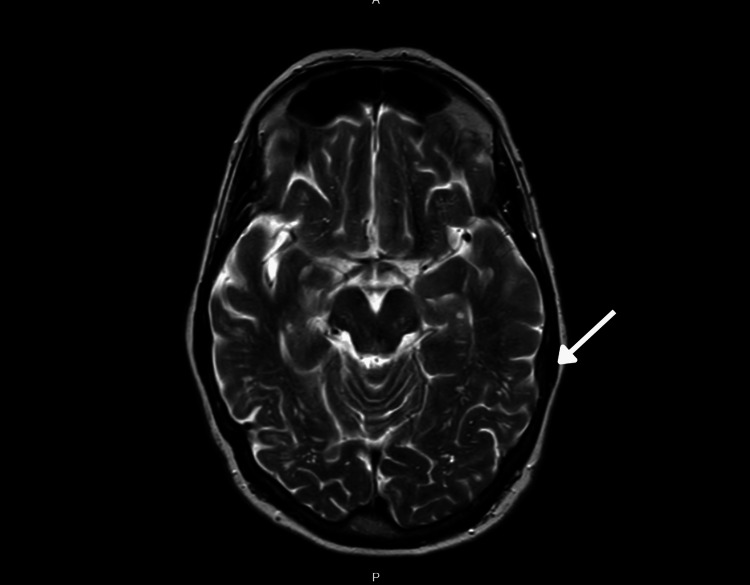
MRI head with contrast done in June 2021 showed resolution of diffuse subcortical white matter changes in the left temporoparietal region. MRI, magnetic resonance imaging
